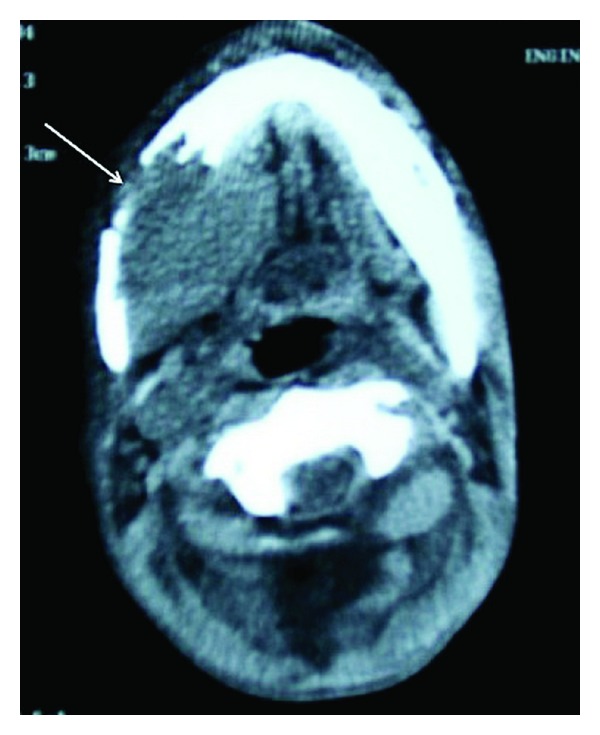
A CT scanning showed a mass with a diameter of 5 cm infiltrating the retromolar and posterior buccal areas with an erosion the mandibular alveolar ridge.
